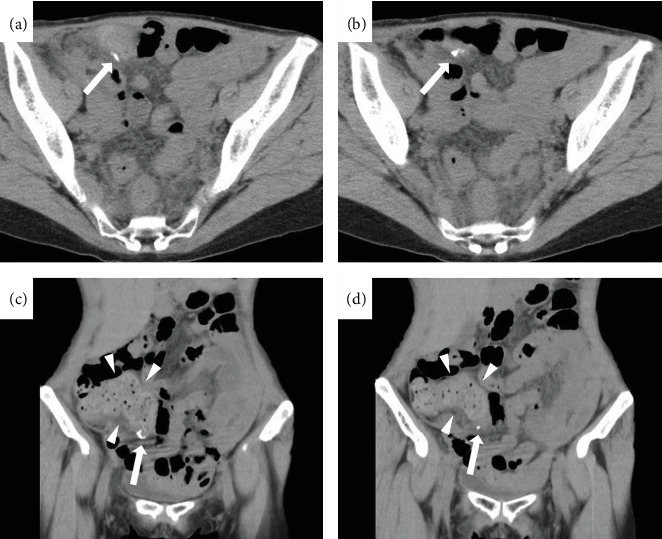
Abdominal CT findings on POD 15. (a–d) Abdominal CT showed no fluid collection around the appendectomy site (arrows), but significant fecal retention in the ileocecal region, including the appendectomy site (arrowheads).
